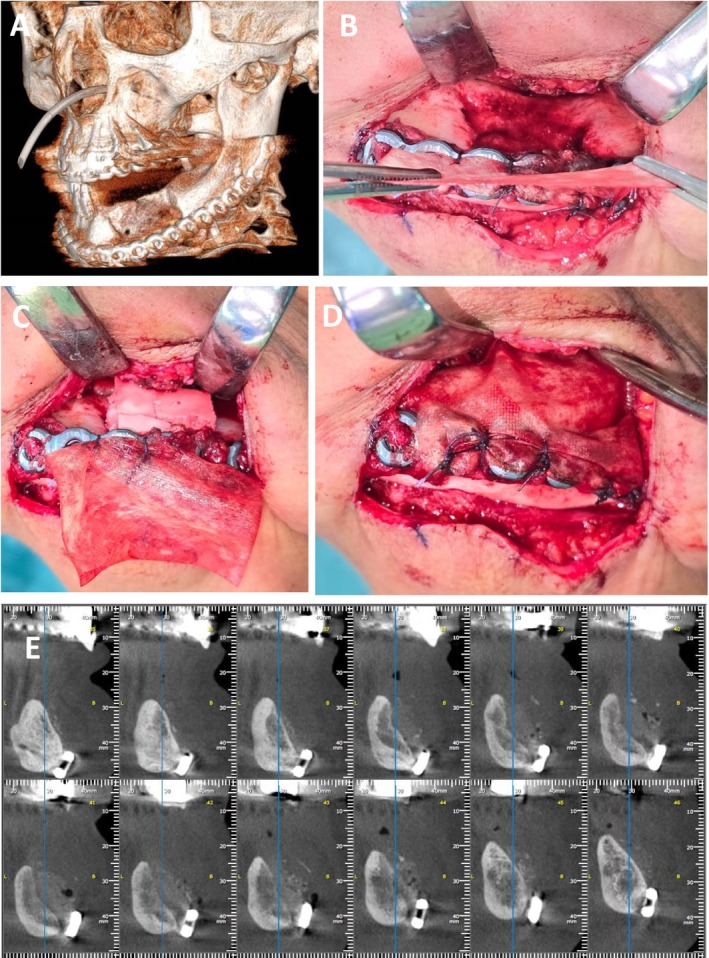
Bone augmentation 9 months following marginal mandibulectomy—Case 1. (A) Three‐dimensional reconstruction from multidetector computed tomography (MDCT) immediately following marginal mandibulectomy and fixation of a reconstruction plate, demonstrating the extent of resection and stabilization. (B) Submandibular surgical approach revealing the reconstruction plate and the bone defect, OSSIX Agile membrane is sutured to the reconstruction plate. (C) The mandibular defect augmented using an autologous bone graft harvested from the anterior iliac crest, in combination with OSSIX Bone. (D) OSSIX Agile membrane covering the bone graft. (E) Eighteen‐month follow‐up Axial CBCT.
